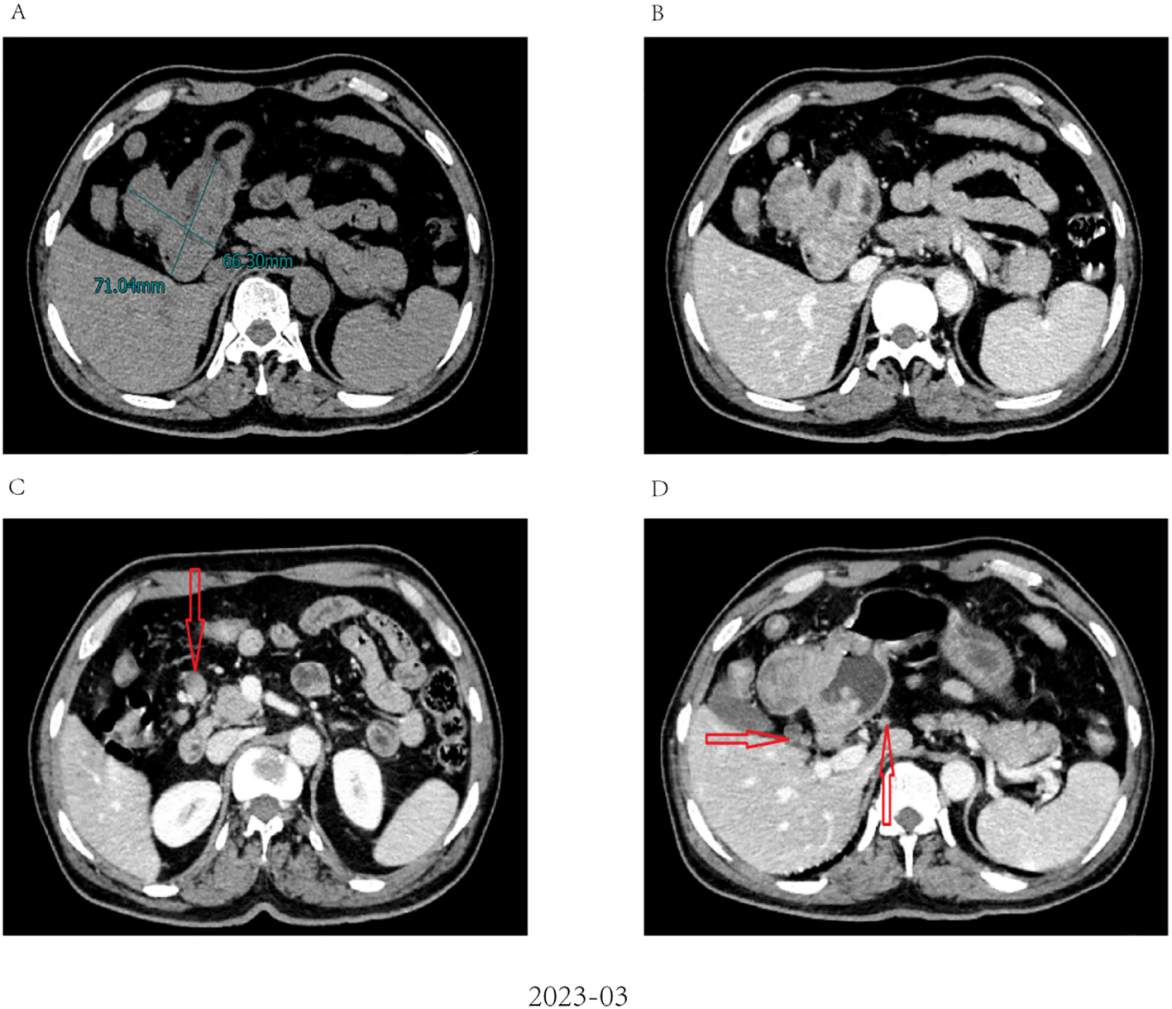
Abdominal CT imaging before chemo-immunotherapy (A). Contrast-enhanced CT revealed a large tumor measuring 71 × 66 mm in size; (B) The wall of the gastric antrum was thickened and enhanced; (C, D) Contrast-enhanced CT revealed multiple enlarged abdominal lymph nodes (red arrow).
